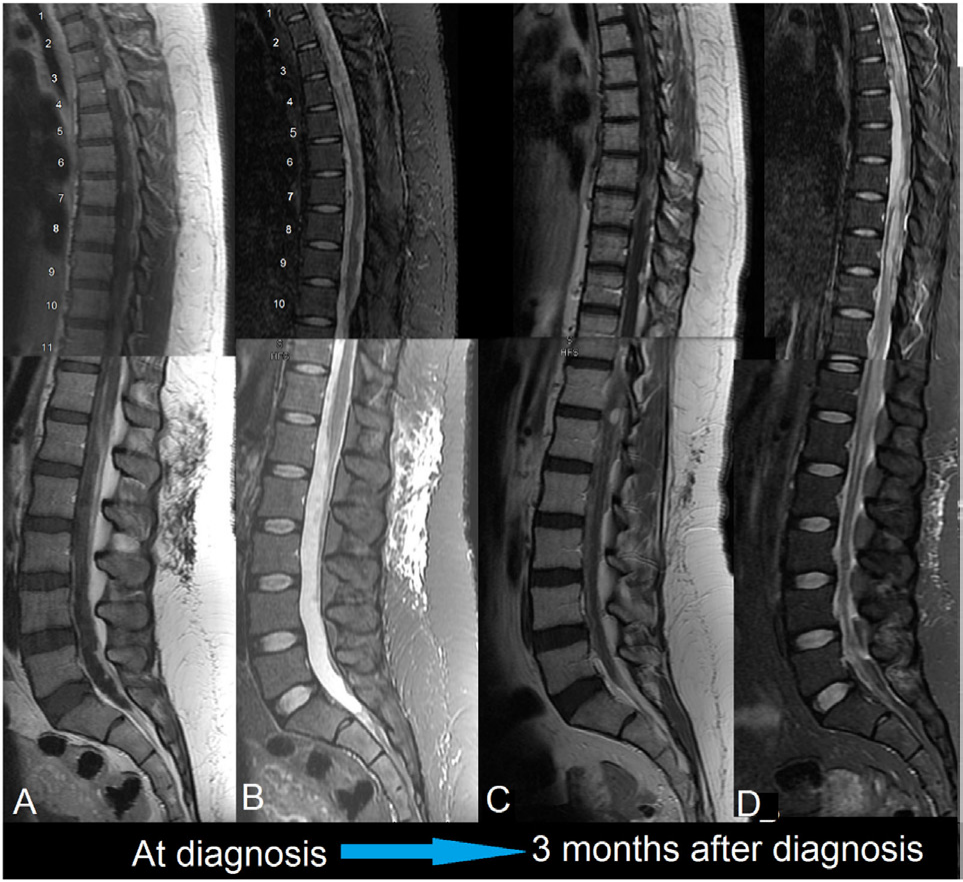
First patient. MRIs of spine, 1.5 T, sagittal. (A) Gd-enhanced: diffuse, nodular enhancement is seen throughout. This is striking dorsally and at the conus medullaris. (B) T2-weighted: the thoracic cord is swollen with intra-medullary edema. This appears to be a reaction to the surrounding leptomeningeal tumor; there may also be an element of cord compression in the thoracic cord. (C) Gd-enhanced: disease in the upper thoracic cord is much improved following radiotherapy. Progression has occurred around the untreated cord caudally. (D) T2-weighted: similarly, intra-medullary edema has improved in the treated areas but worsened elsewhere.
